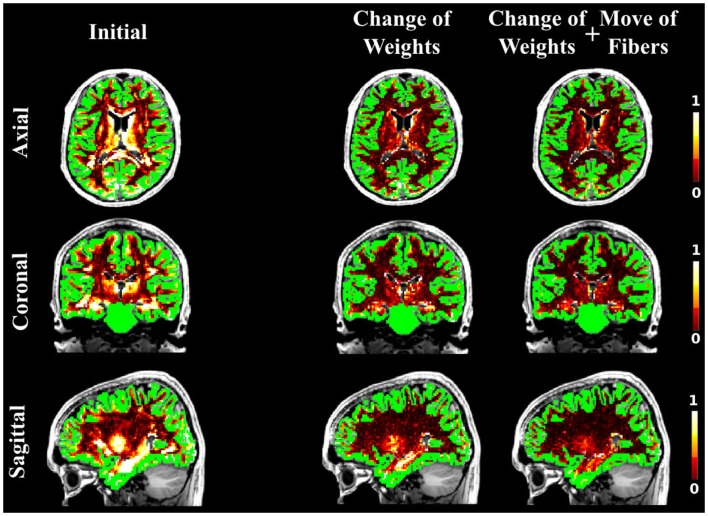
The NMSE maps overlayed onto the T1-weighted and the GM regions. The initial set was estimated using the GIBBS tracking algorithm, which was then used as an initial set for our approach. The results when optimizing the weights only (second column) and when all suggested proposals are used (third column) are compared to the initial set (first column).
